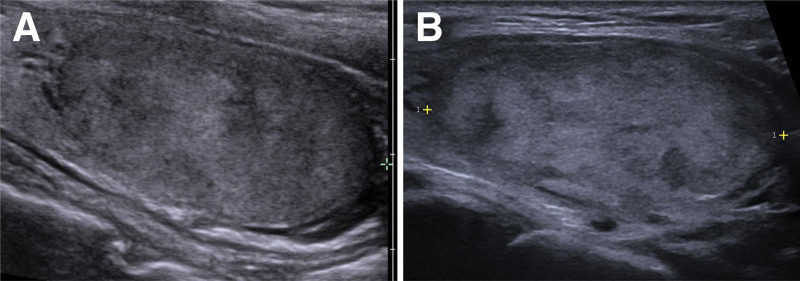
The ultrasonographic examination showed a 3.8-cm left thyroid mass, featuring solid composition, hypoechogenicity, wider-than-taller shape, smooth margin, and absence of echogenic foci categorized as thyroid imaging reporting and data system (TI-RADS) 4 (A) in Aug 2021 (B) in Jun 2023, with no discernible interval change.
